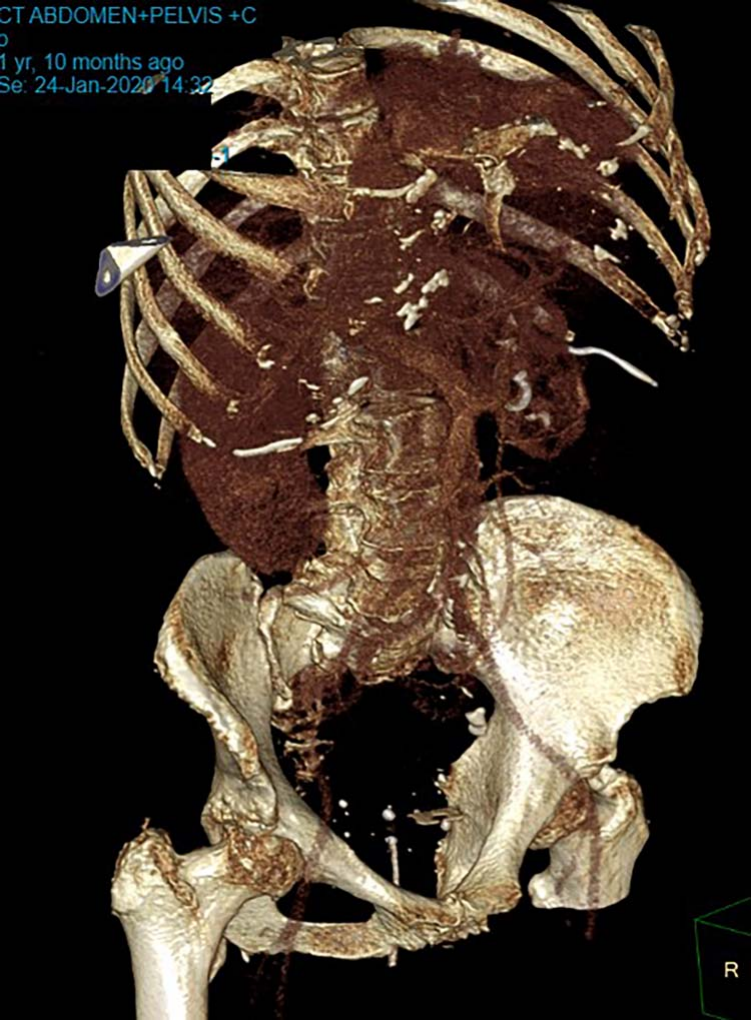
CT reconstructed image, BC seen in lower pelvis.
